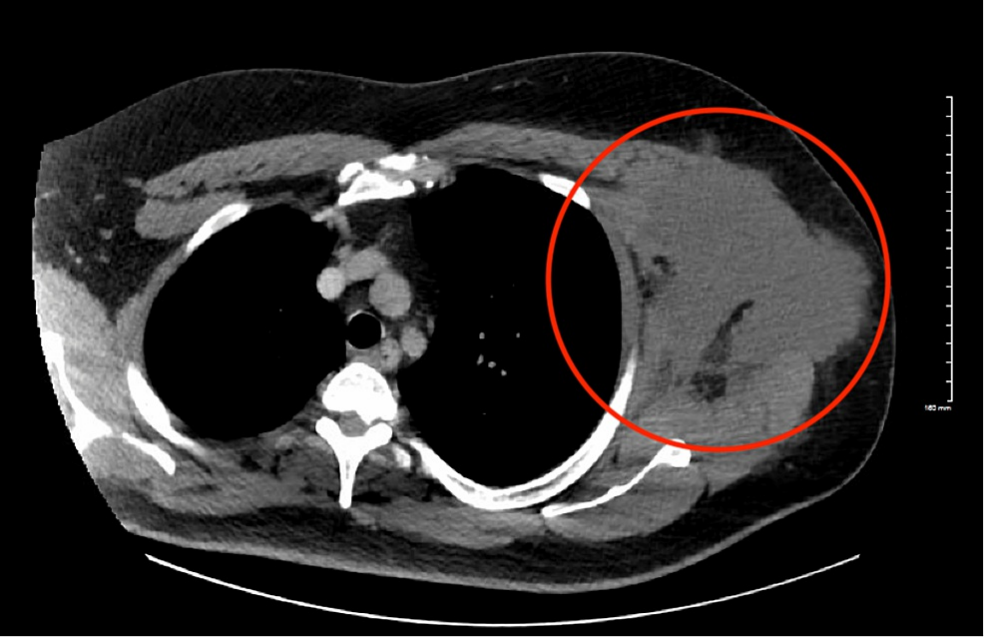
CT (axial view) of the chest showing a large soft tissue density mass (red circle) involving the left chest wall and axillary region measuring 14x10 cm
